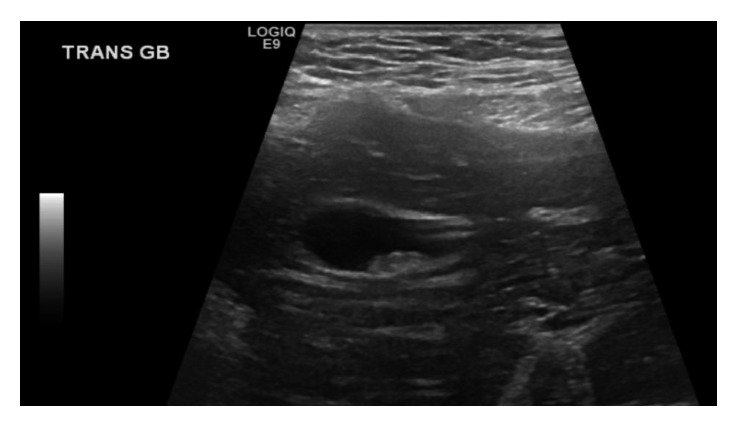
Transabdominal ultrasound demonstrated sludge and stones in a contracted gallbladder.
